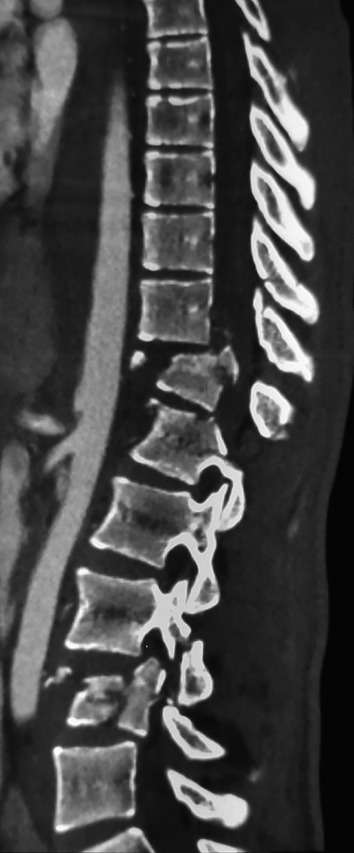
CT scan showing a burst fracture of 12
